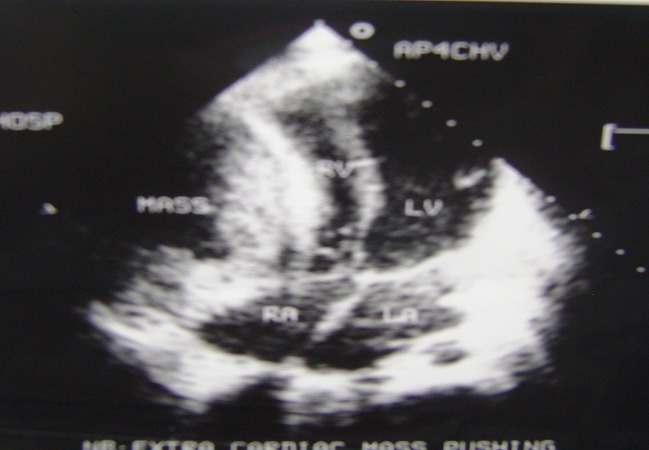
Transthoracic echocardiography showing an echolucent mass compressing the right ventricle
